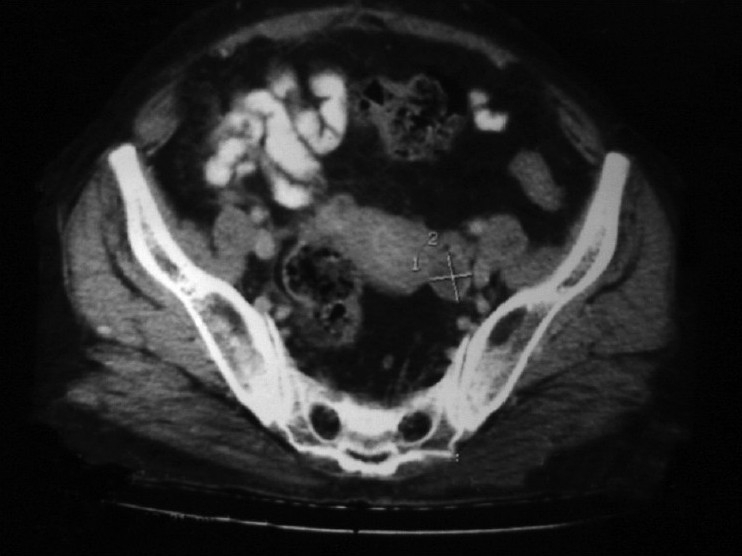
Contrast-enhanced computed tomography scan of the abdomen (pre-treatment) showing a small hypodense cystic lesion in the left adenexal region
